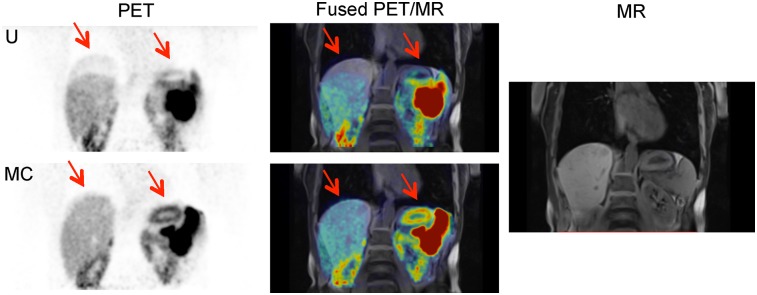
Case 5. Coronal uncorrected (U) and motion-corrected (MC) PET images, along with T1-weighted volumetric interpolated breath-hold examination PET/MR images showing reduction in attenuation misalignment artifacts (arrows), and MR image alone.
